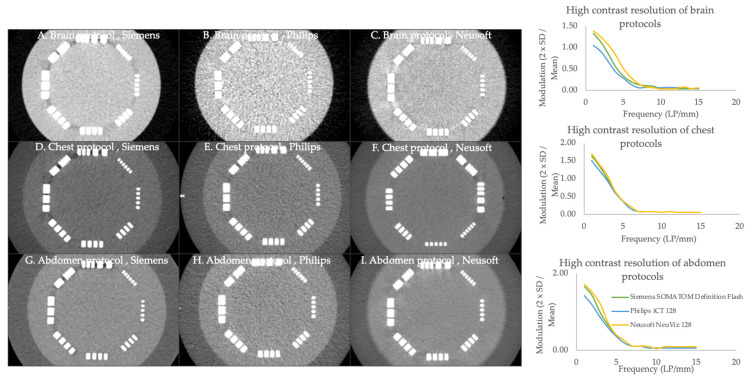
Comparison of high-contrast spatial resolution of brain, chest, and abdomen protocols with various CT scanners. The line pair (shown in the white line) is used to analyze high-contrast spatial resolution. The green, blue, and yellow lines represent the Siemens SOMATOM Definition Flash, Philips iCT128, and Nuesoft NeuViz 128, respectively.
